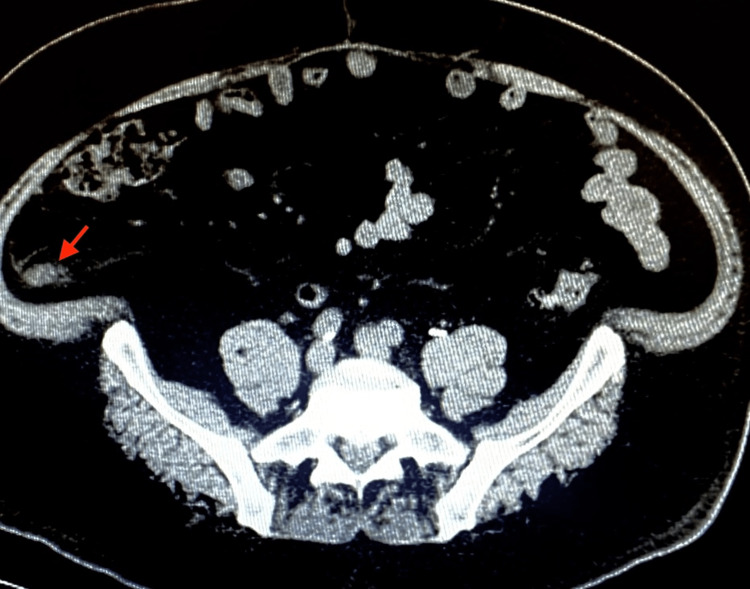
CT of the abdomen. Red arrow: the tip of the appendix reveals minimal inflammation.
